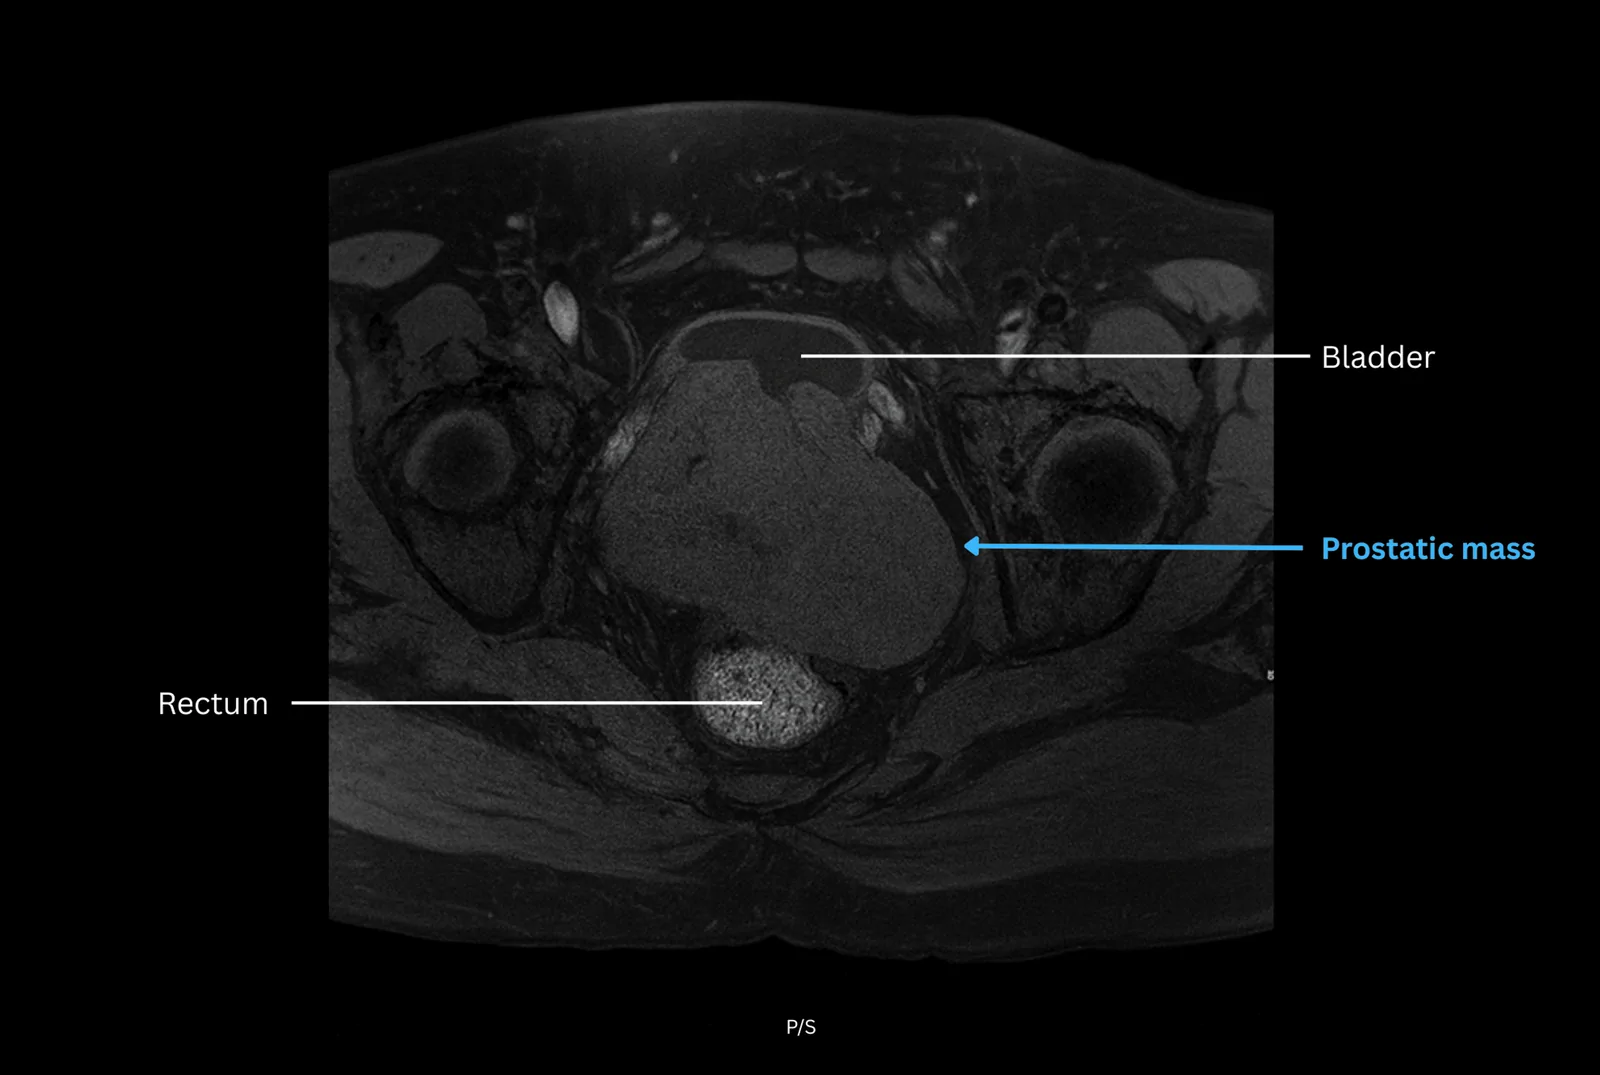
Pelvic MRI showing the prostatic mass and adjacent anatomical structures. The blue arrow indicates the mass, which occupies most of the prostate and nearly obliterates the rectoprostatic fat plane.
